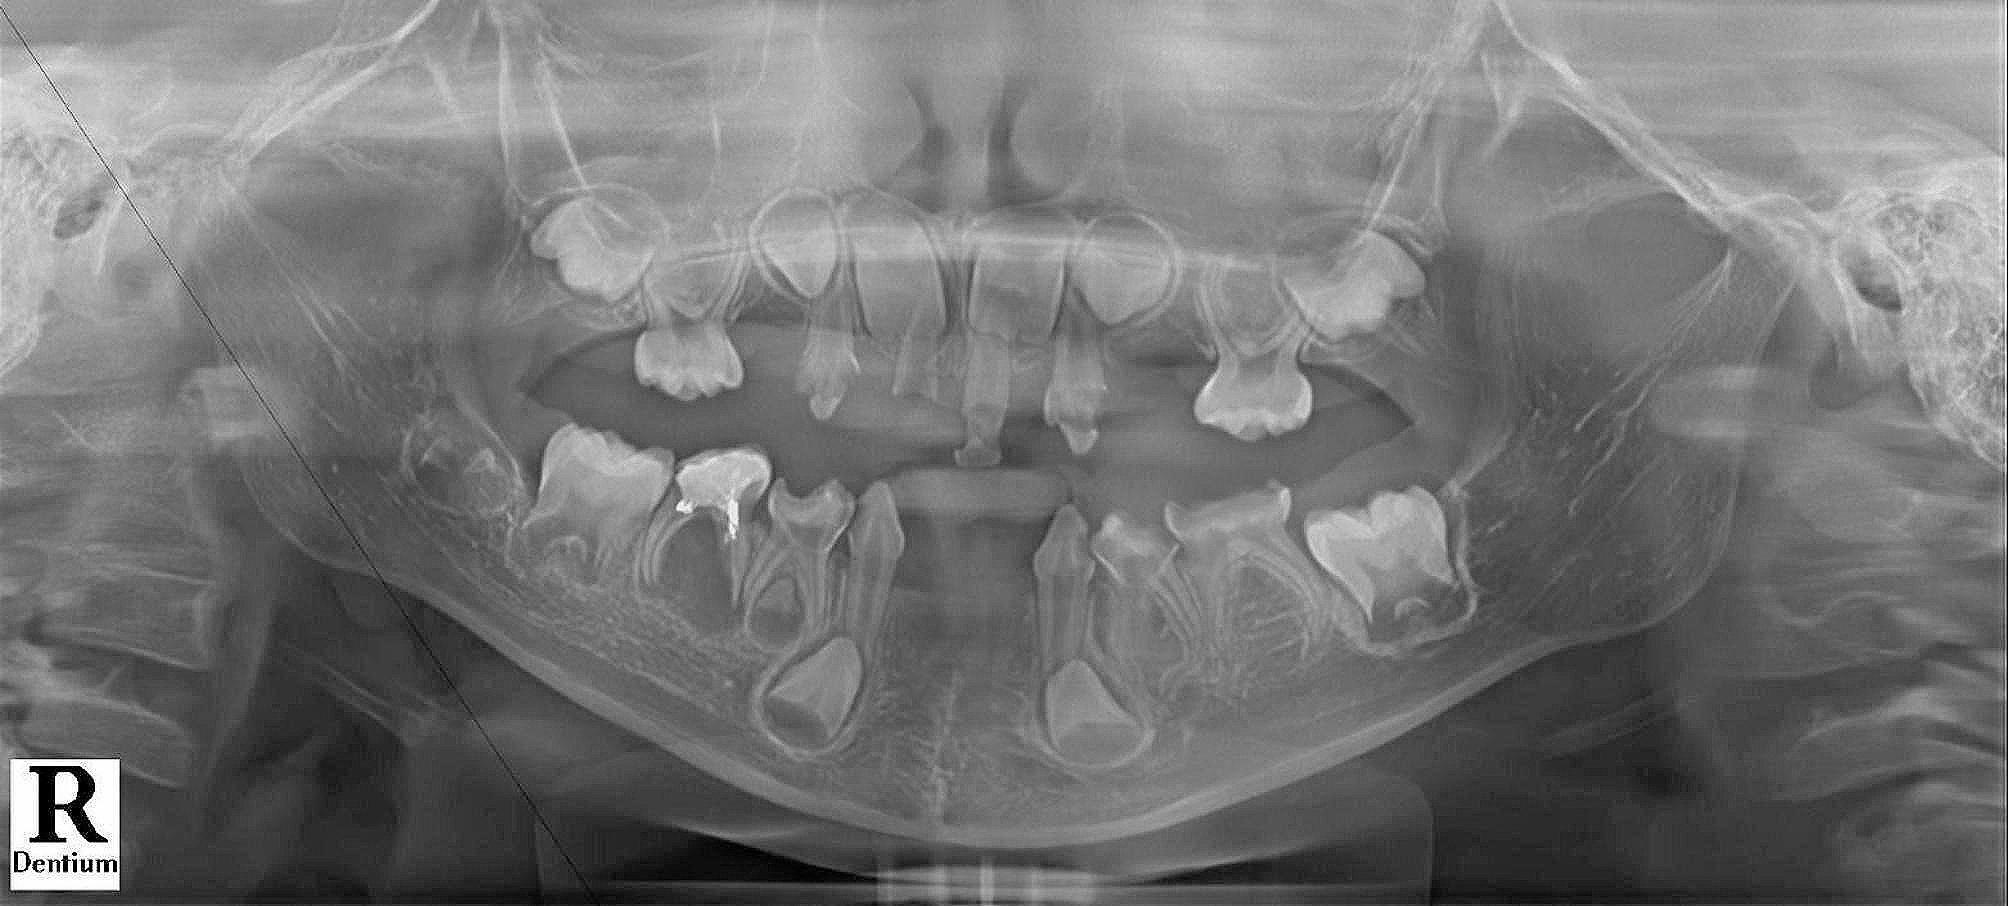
Initial panoramic radiograph of the patient
